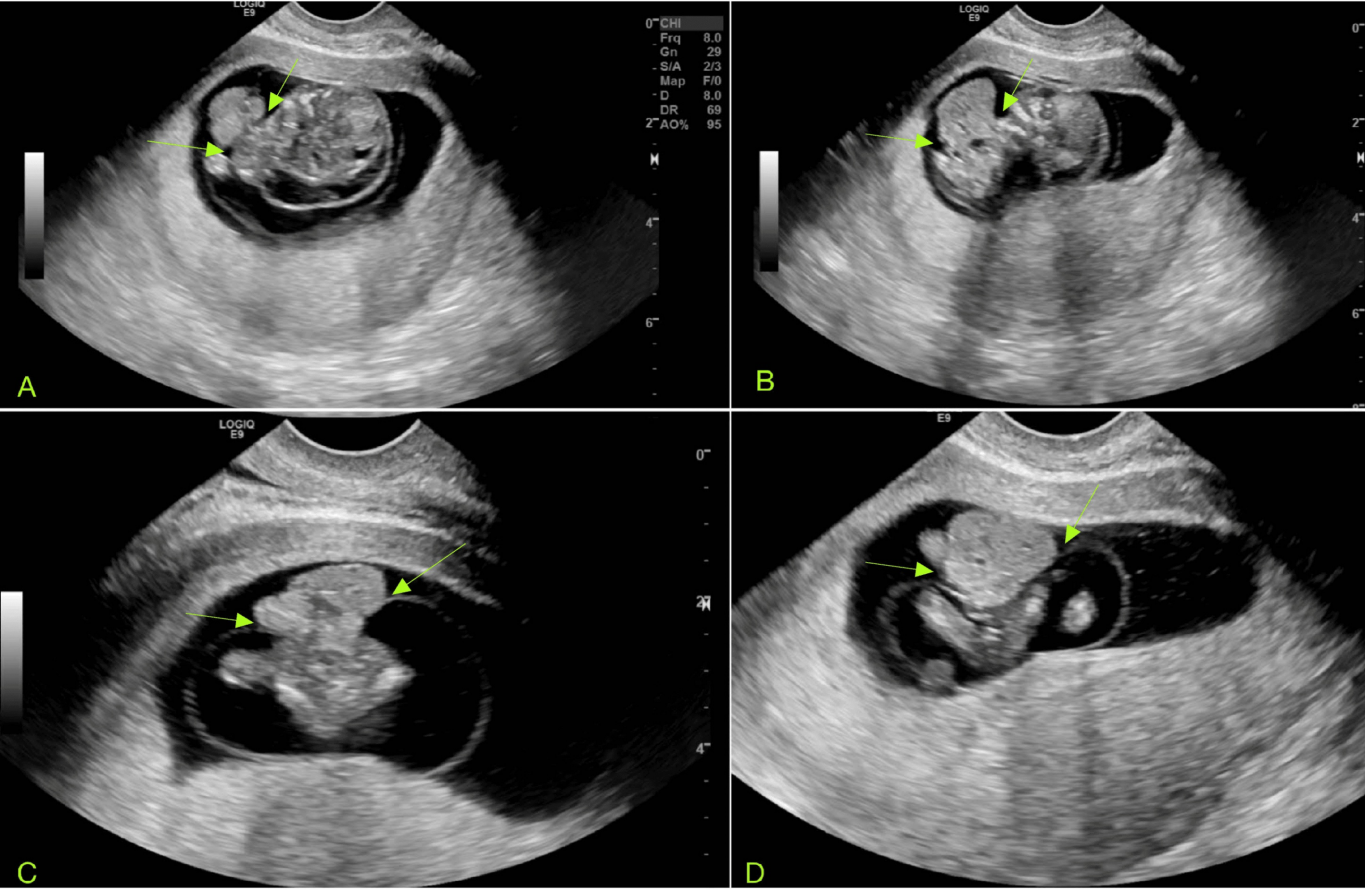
Images A and B display sagittal ultrasound views of the ventral defect (yellow arrows), while Images C and D present transverse views of the same defect (yellow arrows), raising concern for gastroschisis
